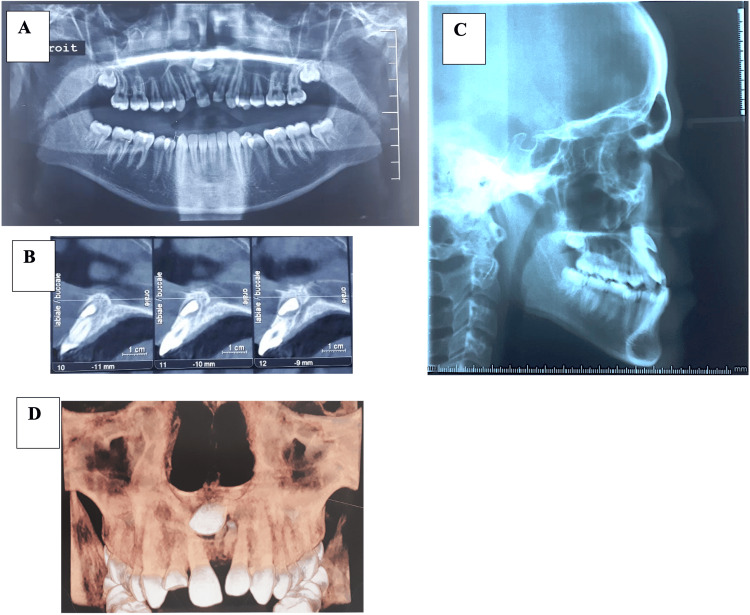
Pre-treatment radiographs (A): Panoramic radiograph, (B): CBCT scans: Cross-sectional slices of the impacted tooth, (C): Lateral cephalometric radiograph, (D): CBCT scan: 3D reconstruction of the maxillary region CBCT: cone beam computed tomography
